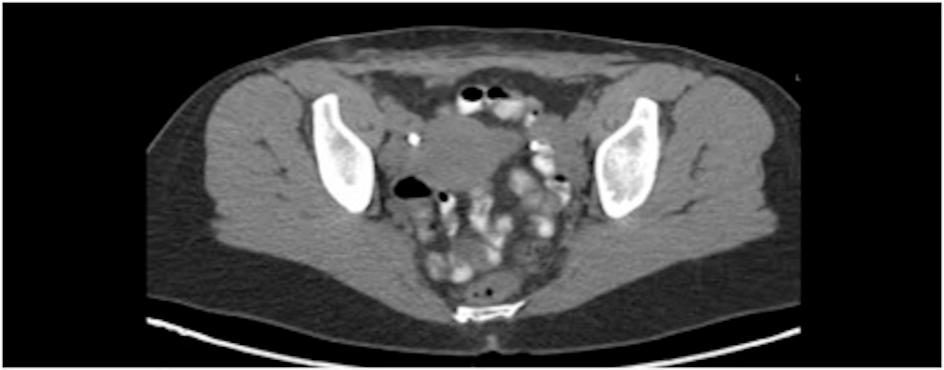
Pelvic CT with abdominal wall soft tissue stranding after tumor resection.
